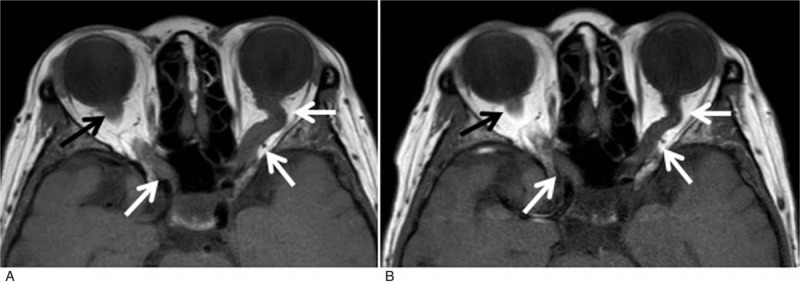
(A) At presentation brain magnetic resonance imaging revealed bilateral swelling of optic nerves extending from the retina to the optic chiasm and swelling of the left optic tract. T1-weighted image in the axial plane shows diffuse enlargement of both optic nerves (arrows). (B) Corresponding T1-weighted image in the axial plane of the same patient 3 months after treatment demonstrates decrease in the size of both nerves.
